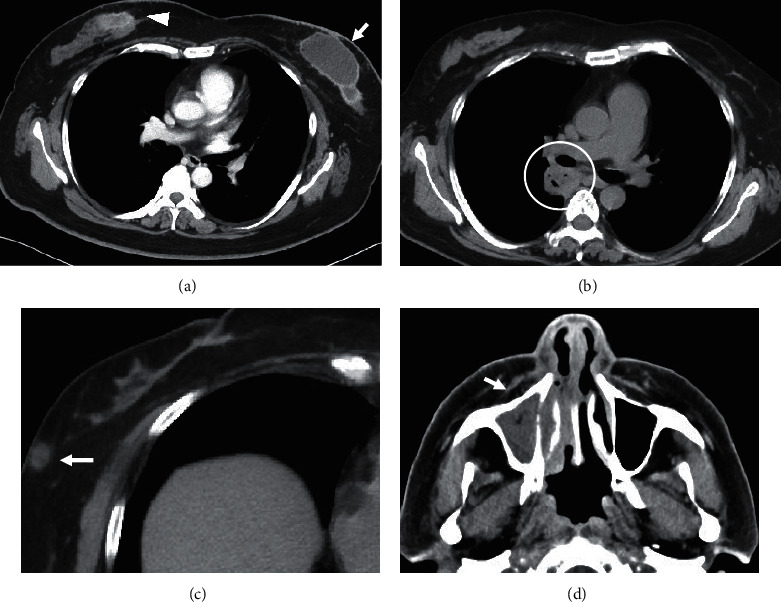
(a) Computed tomography showing tumor-like lesions in the patient's breasts (right, arrowhead; left, arrow) on the first admission. (b) Whole-body computed tomography revealing pulmonary consolidation in the right lower lobe (circle). (c) A small nodule in the right breast (arrow), and (d) recurrence of right maxillary sinusitis (arrow) during the second admission.
